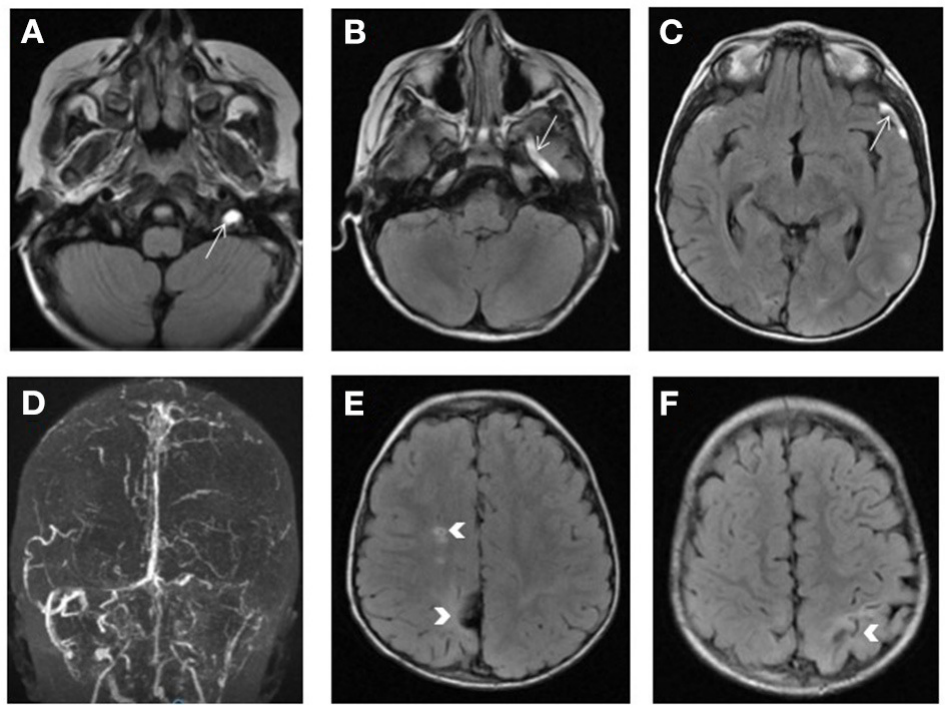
Homocystinuria. Bright signal of the left transverse and sigmoid sinuses as well as the left jugular vein, superficial middle cerebral vein (arrow) and vein of Labbe on FLAIR weighted images (A–C) consistent with venous thrombosis and confirmed by the loss of the normal flow signal on 2D TOF venogram (D). Bilateral areas of encephalomalacia involving the parietal lobes with lacunar infarct in the right centrum semiovale (arrows in E,F).
